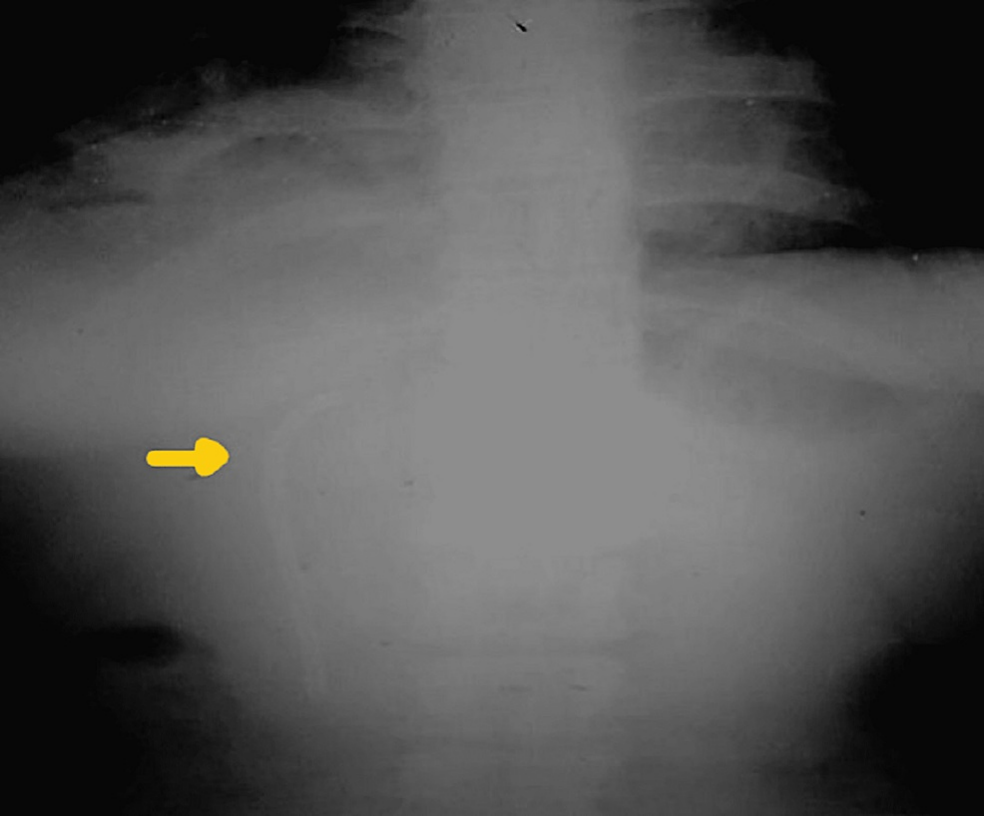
X-ray of the erect abdomen showing the CBD stent insitu. CBD- Common bile duct
